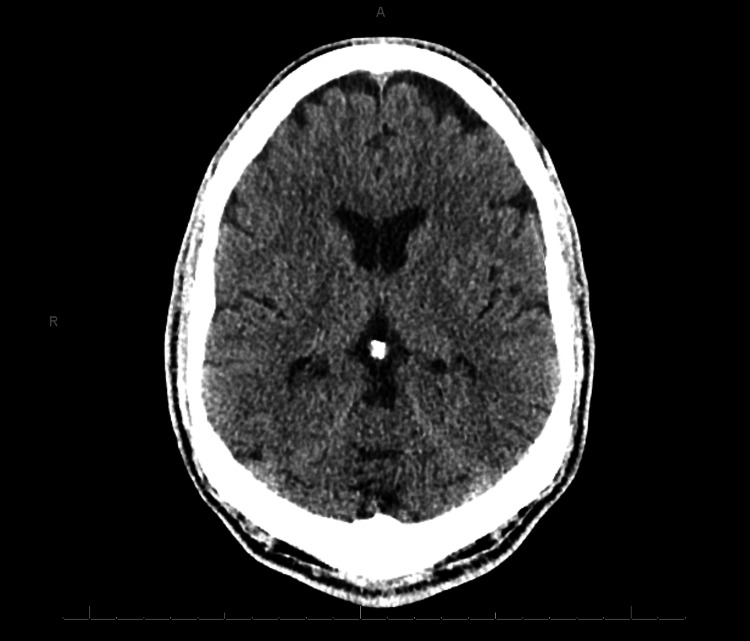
CT-head non-contrast of the patient Radiology described involutionary changes over the prefrontal cortex.
